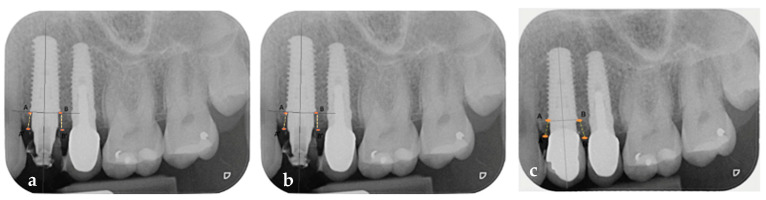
(a) Mesial and distal bone peak measurement on periapical radiograph taken at T1. (b) Mesial and distal bone peak measurement on periapical radiograph taken at T2. (c) Mesial and distal bone peak measurement on periapical radiograph taken at T3. A-A′ represents the measurement of the mesial bone peak to the implant, defined as the vertical distance between the most coronal point of the mesial alveolar bone adjacent to the implant and the line passing through the head of the implant. B-B′ represents the measurement of the distal bone peak to the implant, defined as the vertical distance between the most coronal point of the distal alveolar bone adjacent to the implant and the line passing through the head of the implant.
